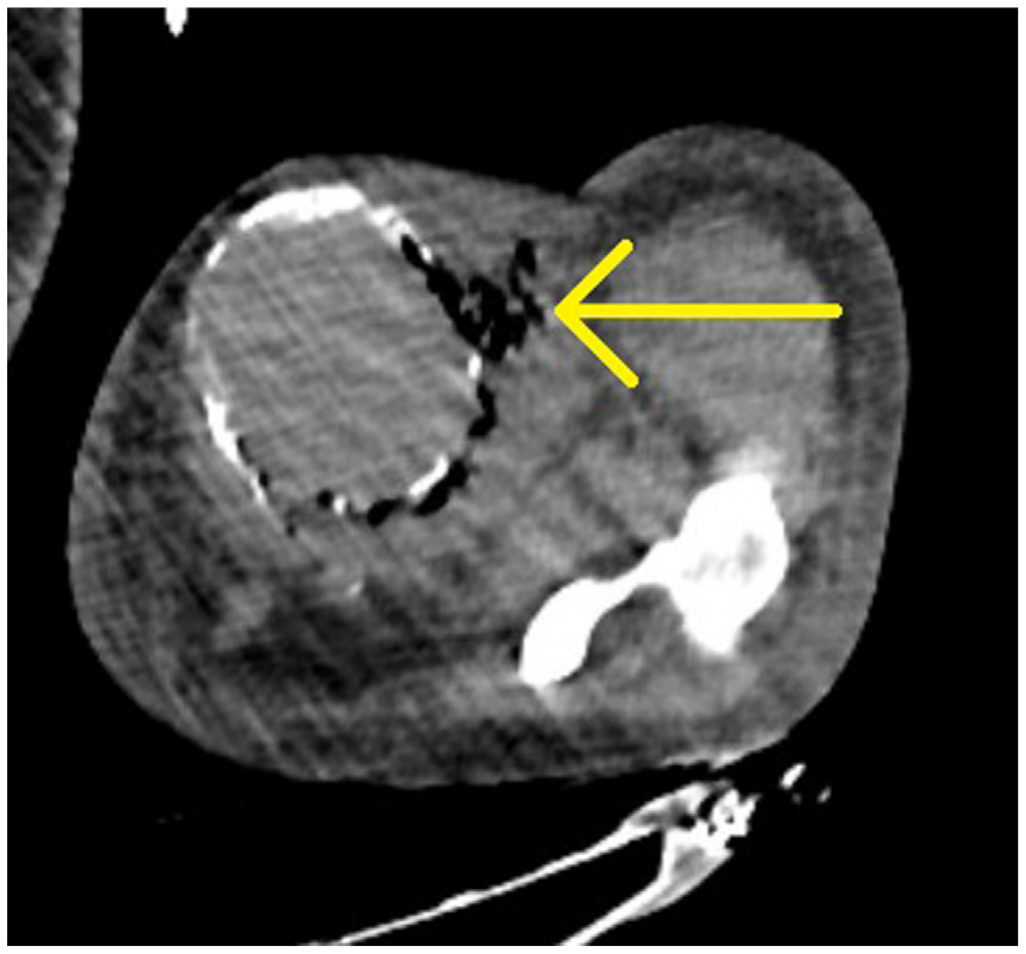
Axial cut from computed tomography done on admission showing gas and fluid surrounding the patient's left upper extremity arteriovenous fistula (AVF). Yellow arrow showing fluid/air collection.
